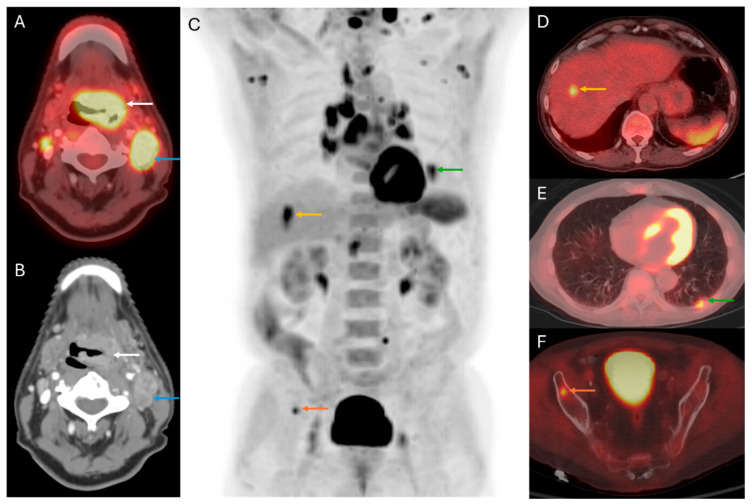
Distant disease detection and Prognosis: 74-year-old male with base of tongue SCC. Axial fused PET/CT (A,D–F), axial CT (B), and coronal MIP (C) images of the staging 18F-FDG PET/CT demonstrate the primary tumor in the tongue base (white arrows), cervical nodal metastases (blue arrows), hepatic (yellow arrows), pulmonary (green arrows), and osseous (orange arrows) metastases. Despite systemic therapy, the patient succumbed to the disease approximately 1.5 years after the examination.
